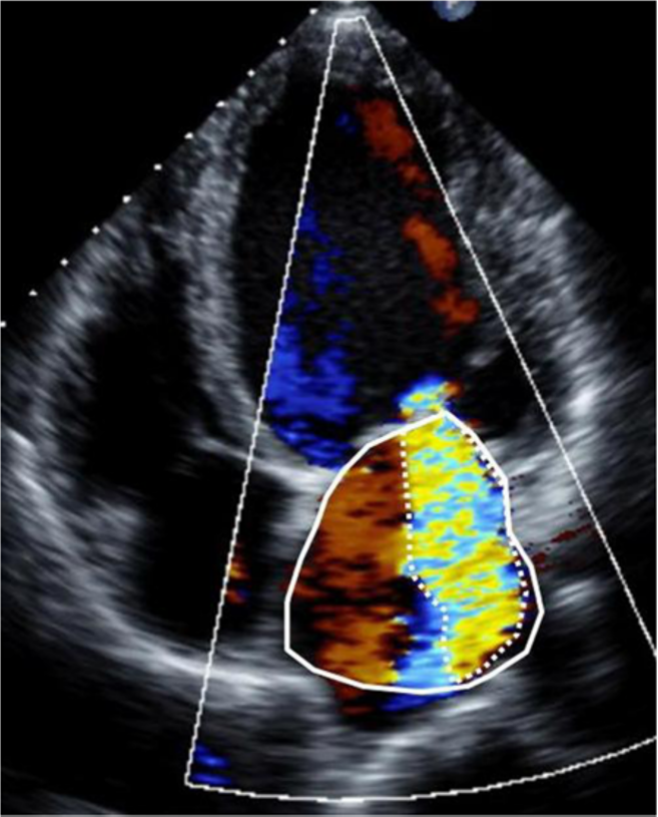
MR quantification by ratio of maximal distal jet area to left atrial area. Assessment of MR severity by distal jet area involves tracing the jet area (dashed white line) in the apical four chamber view and comparing the ratio of jet area to the left atrial area (solid white line). See Tables 3 and 4 for MR severity grades corresponding to different jet area:left atrial area ratios.
